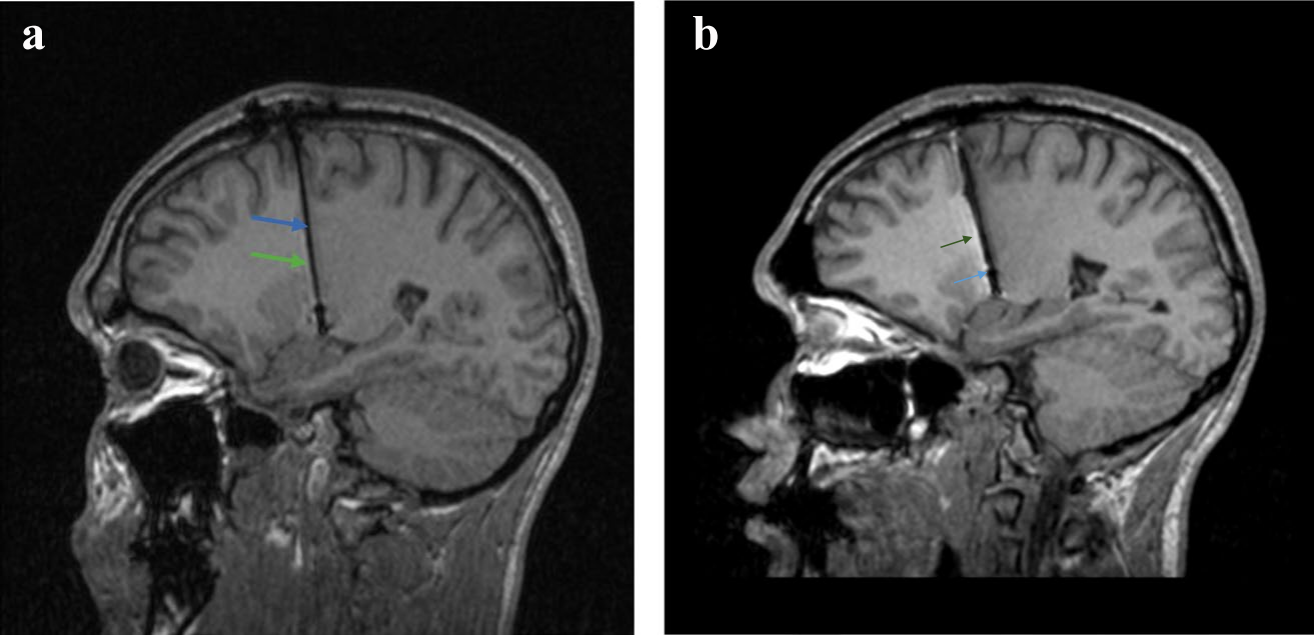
MRI images of the brain- T1, sagittal section, one year after electrode implantation. Normal postoperative findings. a right electrode (blue arrow - electrode, green arrow - artifact – thin white border around the electrode). b Left electrode (blue arrow - electrode, green arrow - artifact - distinctive white border around the electrode)
